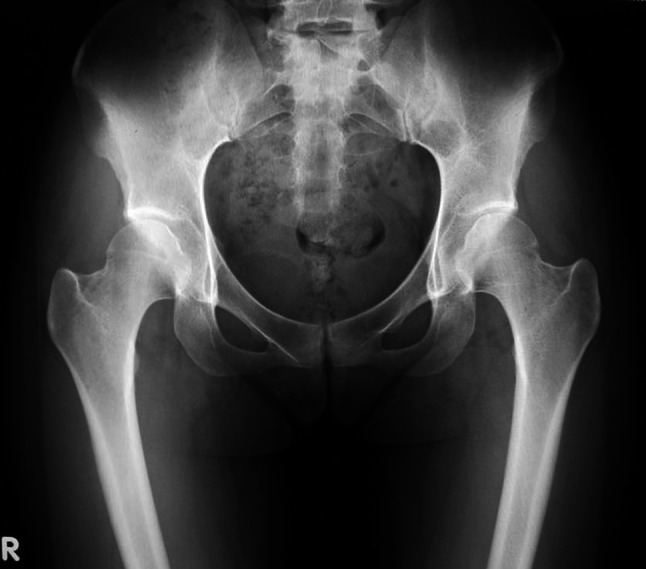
The preoperative anterior–posterior radiograph demonstrated crossover sign at the left acetabulum indicating acetabular retroversion
